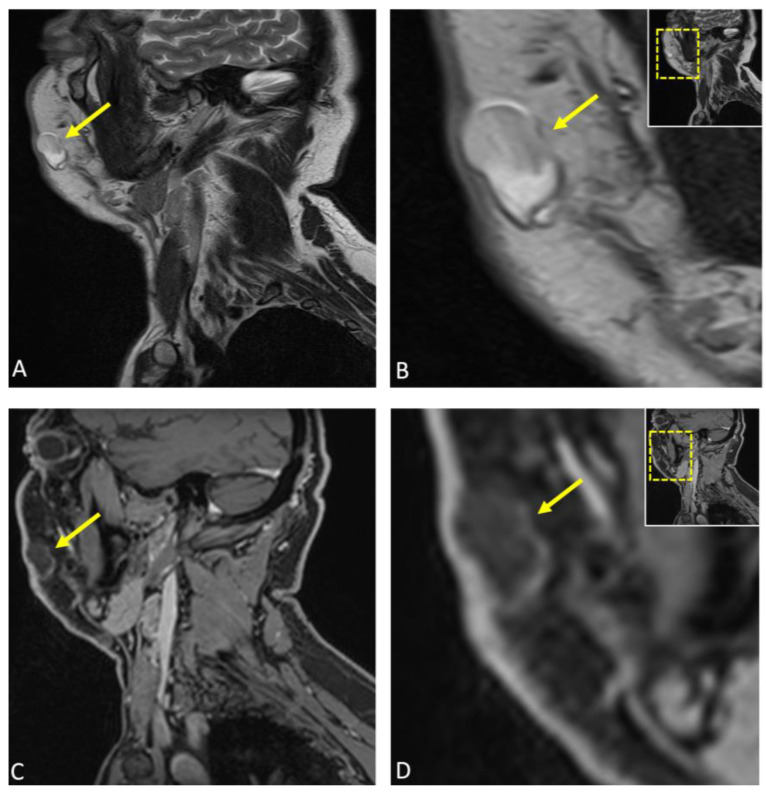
Subsequently, considering the patient’s age and medical history, a comprehensive diagnostic imaging workup was conducted, encompassing both conventional and dental MRI protocols for visualizing the facial skull and suprahyoidal neck using a contrast agent. This approach was undertaken with the objective of attaining a more detailed understanding of the patho-anatomy within the specified region, delineating soft tissues, and characterizing the lesion’s internal composition. The patient underwent MRI at 3 Tesla (MAGNETOM Skyra, release VE11E, Siemens Healthineers, Erlangen, Germany) with the following MRI protocols: Sagittal reconstructions of the lesion are presented in (A) T2-weighted turbo spin echo (TSE) and (C) T1-weighted Dixon MRI, with an enlarged view in (B,D), respectively. The lesion (arrow) measured 24 × 12 × 16 mm and was identified as a well-circumscribed macrocystic mass within the subcutaneous fat of the right cheek, specifically located in the superficial fascia. Mild compression of the levator anguli oris muscle was observed, with broad-based contact between the lesion and the overlying skin. No significant contrast enhancement was noted in the lesion’s capsule, although there was a slight increase in protein concentration and isotropic diffusion restriction, findings consistent with those observed in epidermoid cysts. Perilesional edema was not present. The rest of the examination revealed no abnormalities in the depicted anatomical areas.
